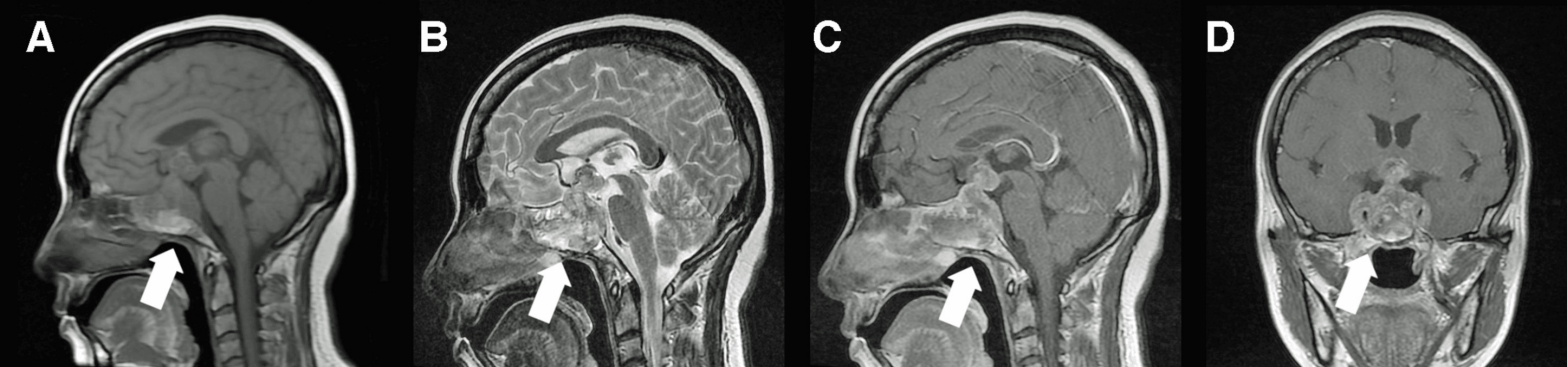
Preoperative MRI A and B: T1-weighted (A) and T2-weighted (B) sagittal MRI scans showing a mass lesion extending from the sphenoid sinus to the suprasellar region (arrows). C and D: Gadolinium-enhanced sagittal (C) and coronal (D) MRI scans showing increased mass enhancement with cavernous sinus invasion (arrows). MRI: magnetic resonance imaging
